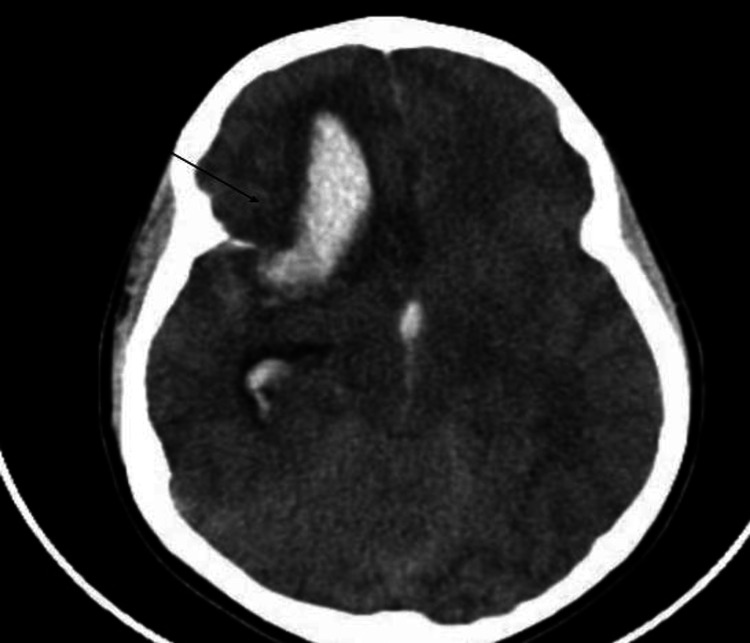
Head CT scan without contrast shows a ​​6.0 x 3.2 x 3.0 cm right frontal intracerebral hematoma (arrow) with rupture into the frontal ventricular horn extending to all ventricles
